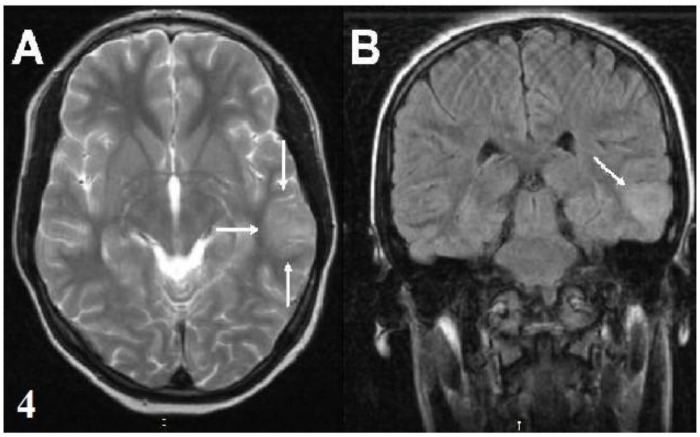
(A,B) The hyperintense cortical lesion in the left temporal region on a T2-weighted axial image and a FLAIR coronal image (Patient 15).
